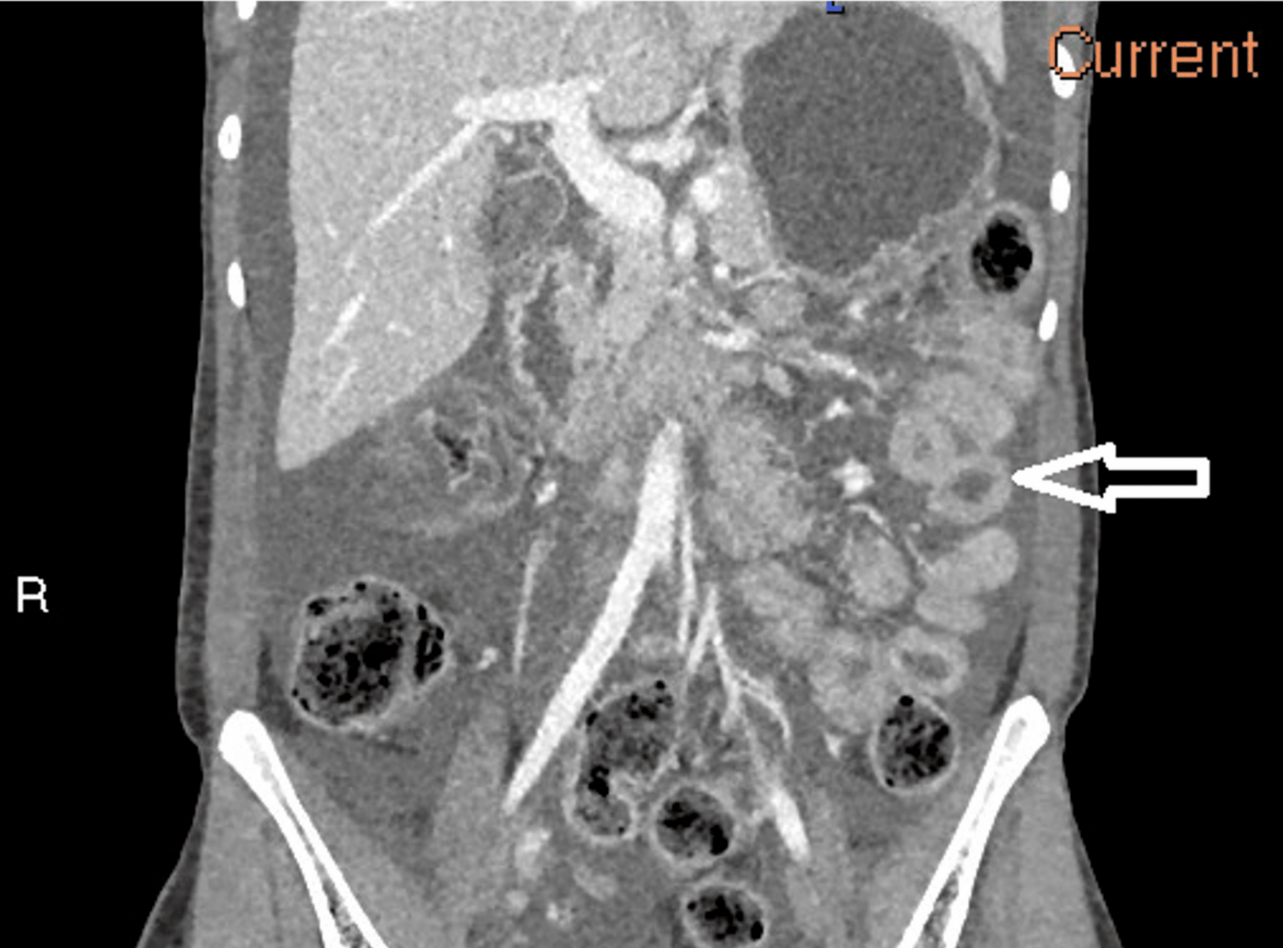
Contrast-enhanced CT of the abdomen and pelvis in the coronal plane demonstrating the “target sign” Coronal cross-sectional images of the abdomen and pelvis show the jejunum (arrow) has circumferential inflammatory thickening of the bowel wall and central hypoattenuation in keeping with oedematous change of the submucosal layer. This manifests radiologically as concentric rings of alternating attenuation, resembling a target, demonstrating the “target sign” (or “double halo sign”).
